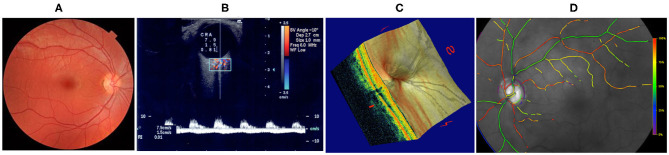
Techniques for non-invasive ocular measurements (Guidoboni et al., 2013). (A) Fundus camera; (B) Color Doppler Imaging; (C) Fourier-Domain Optical Coherence Tomography (FD-OCT); (D) Retinal Oximetry. Images reproduced from Guidoboni et al. (2013), with permission.
